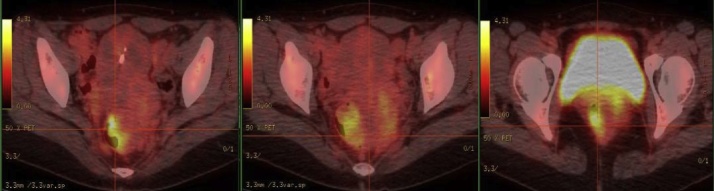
PET-CT showed 18FDG uptake of the rectal endometriosis.
